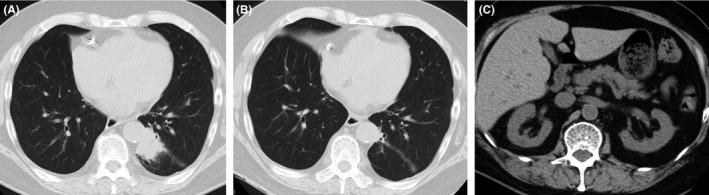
Chest and abdominal computer tomography (CT). (A) Chest CT in March X years showed an increase in tumor size in the left lower lobe. (B) Chest CT on June X showed a reduction in tumor size. (C) No pancreatic tumor showed on abdominal CT in June X
